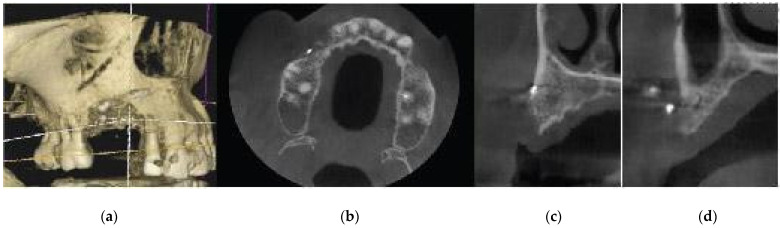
Pre-op CBCT situation—(a) 3D representation of the defect, (b) axial upper maxilla image, (c) cross-sectional image site 13, (d) cross-sectional image site 14.
